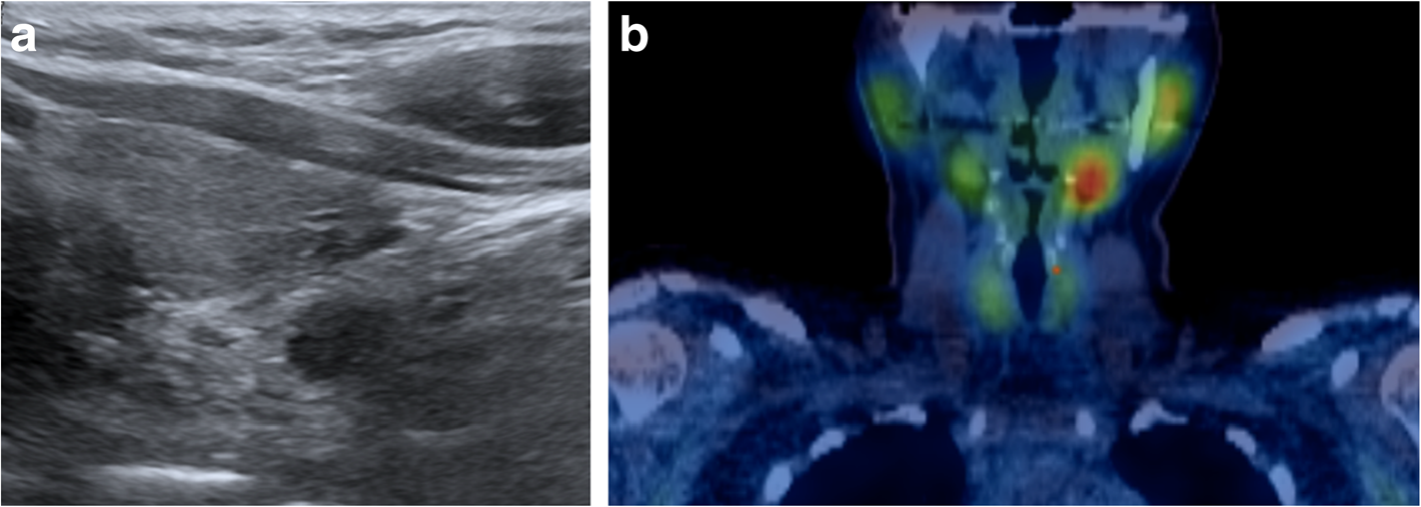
a, b Ultrasound and coronal view SPECT/CT of the thyroid and hyperparathyroid in the 32-year-old female patient showed a 9-mm hypoechoic nodule with high uptake in the area of the left inferior parathyroid and localising a parathyroid adenoma
